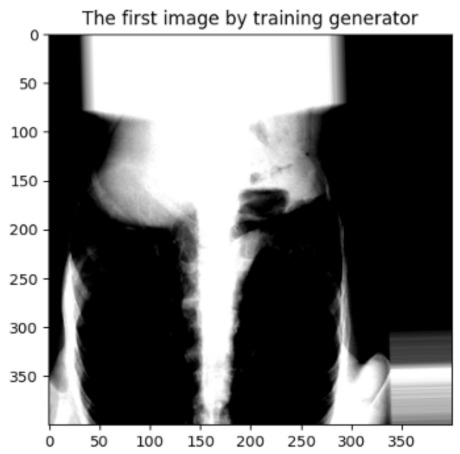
A chest X-ray image with data augmentation.
